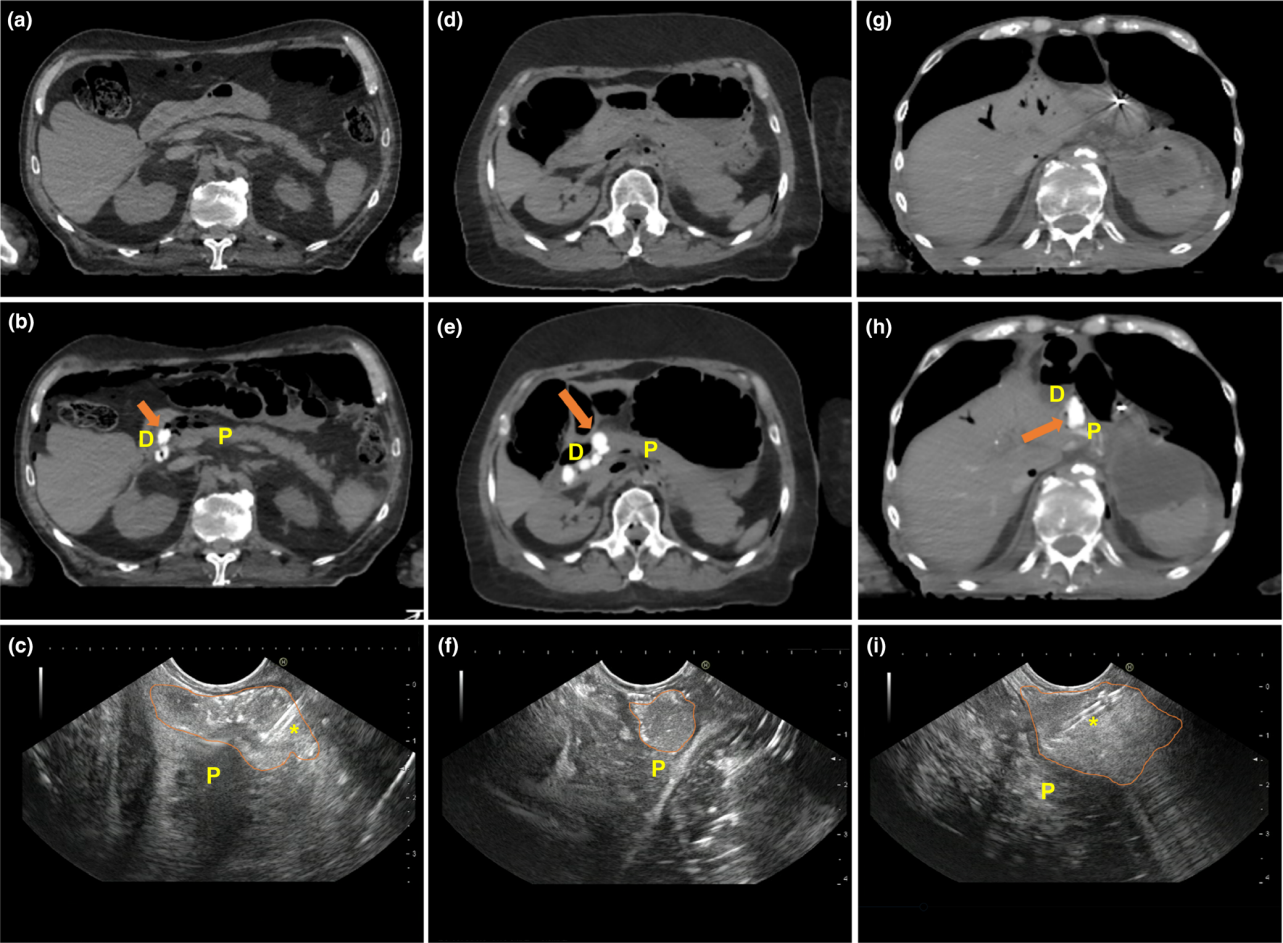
Pre‐ and post‐CT and endoscopic ultrasound (EUS) images of polyethylene glycol (PEG) hydrogel injection. (a) Pre‐injection CT on Cadaver 1. (b) Post‐injection CT on Cadaver 1, the orange arrow indicates injected PEG hydrogel. (c) EUS imaging during PEG hydrogel injection on Cadaver 1, the orange region delineates injected PEG hydrogel. (d) Pre‐injection CT on Cadaver 2. (e) Post‐injection CT on Cadaver 2, the orange arrow indicates injected PEG hydrogel. (f) EUS imaging during PEG hydrogel injection on Cadaver 2, the orange region delineates injected PEG hydrogel. (g) Pre‐injection CT on Cadaver 3. (h) Post‐injection CT on Cadaver 3, the orange arrow indicates injected PEG hydrogel. (i) EUS imaging during PEG hydrogel injection on Cadaver 3, the orange region delineates injected PEG hydrogel. (Abbreviations; P, Pancreas, D, Duodenum, Asterisk, 19G FNA needle.).
